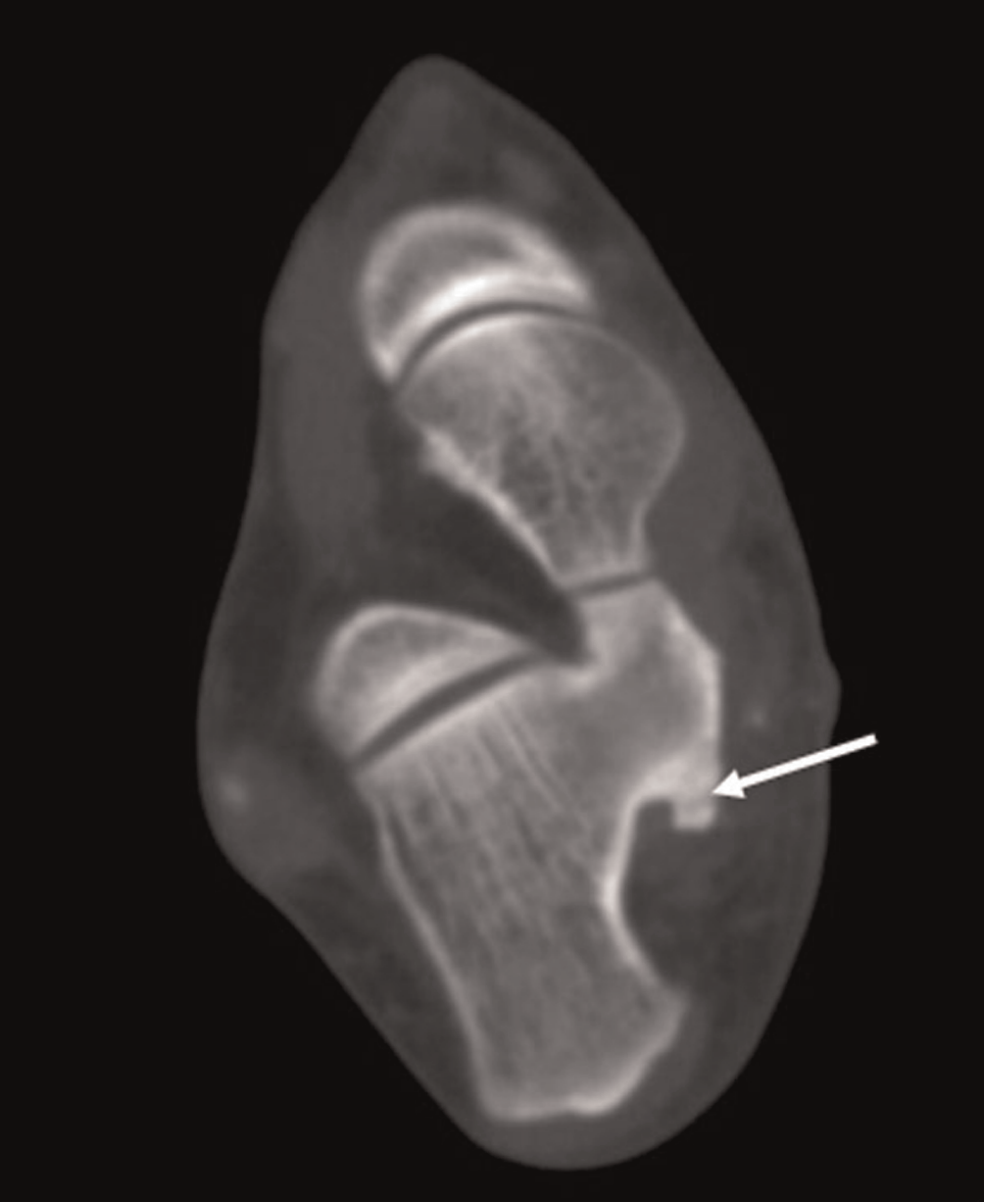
Axial CT scan taken at the 1-month postoperative of the foot shows an exofitic lesion (arrow) detected posteromedially to the calcaneus (5 × 3 mm in size).
